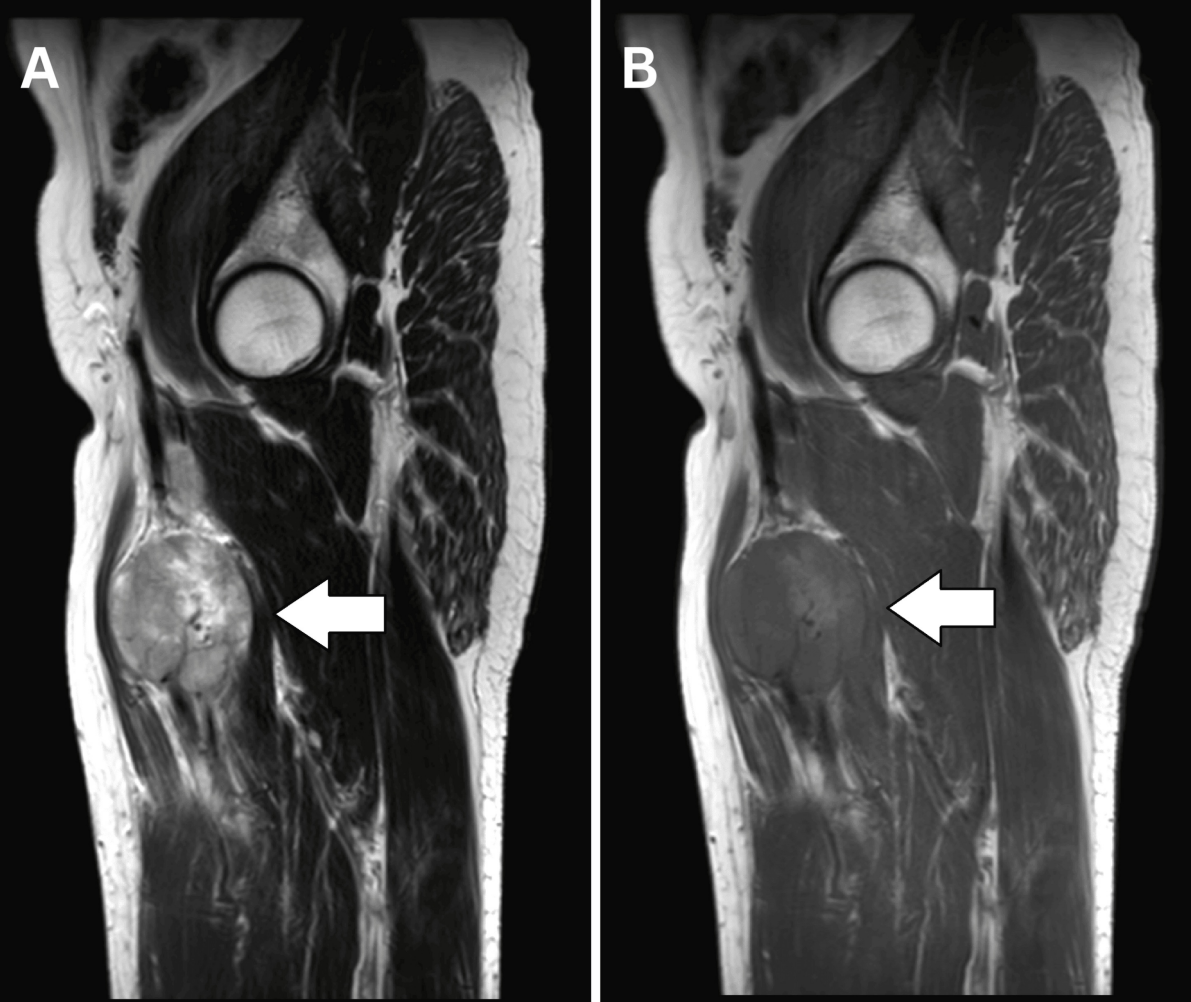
Magnetic resonance imaging (MRI) of femoral vein leiomyosarcoma. A) Sagittal T2 weighted image shows a well-defined mass lesion appearing hyperintense (white arrow); B) Sagittal T1 weighted images show a well-defined mass lesion appearing iso-intense imaging (white arrow). Lesion lies between adductor magnus medially vastus intermedius and rectus femoral laterally.
